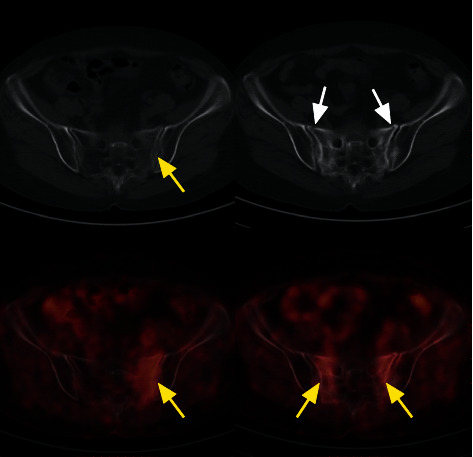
A 71-year-old woman who underwent pelvic radiotherapy for cervical cancer developed hip pain at 9 months after radiotherapy. Diffuse, mild, linear FDG uptake (SUVmax, 3.2) parallel to the sacroiliac joint in the left sacral ala (yellow arrow) was found in the fused axial image, while the low-dose CT image was normal. Follow-up FDG PET/CT at 12 months after diagnosis of SIF. The SIF became bilateral and showed FDG uptake (left SUVmax, 2.6; right SUVmax, 2.4); the low-dose CT image showed increased bone density and a new anterior sacral fracture line (white arrow).
